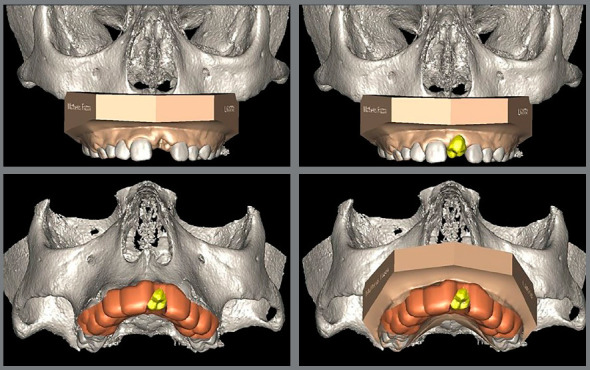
Superimposition of maxillary CBCT, intraoral scan and segmented dental element chosen, for the construction of the surgical guide.
